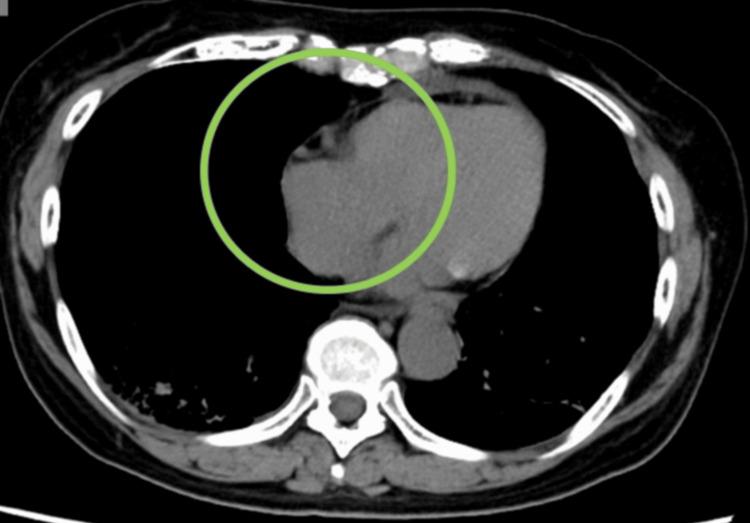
A CT scan taken three months later confirmed the disappearance of the right atrial tumor
